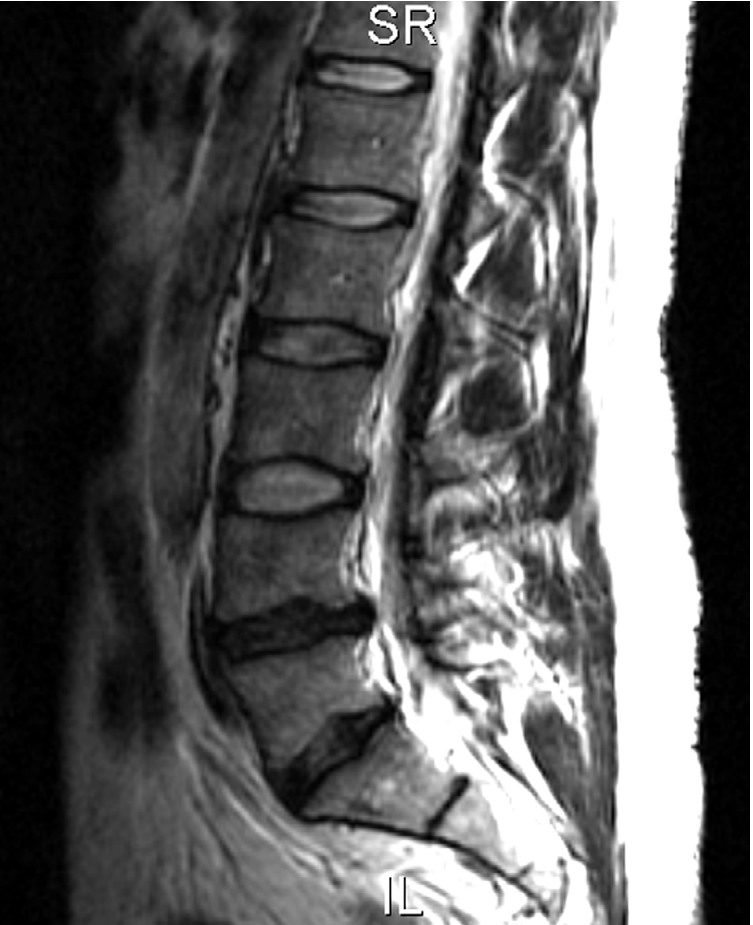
T2 weighted sagittal MRI demonstrating a loss of normal height and signal involving the L4–L5 disc and a broad-based paracentral disc bulge that contacted the thecal sac (arrow). L5-S1 disc degeneration was also present.
